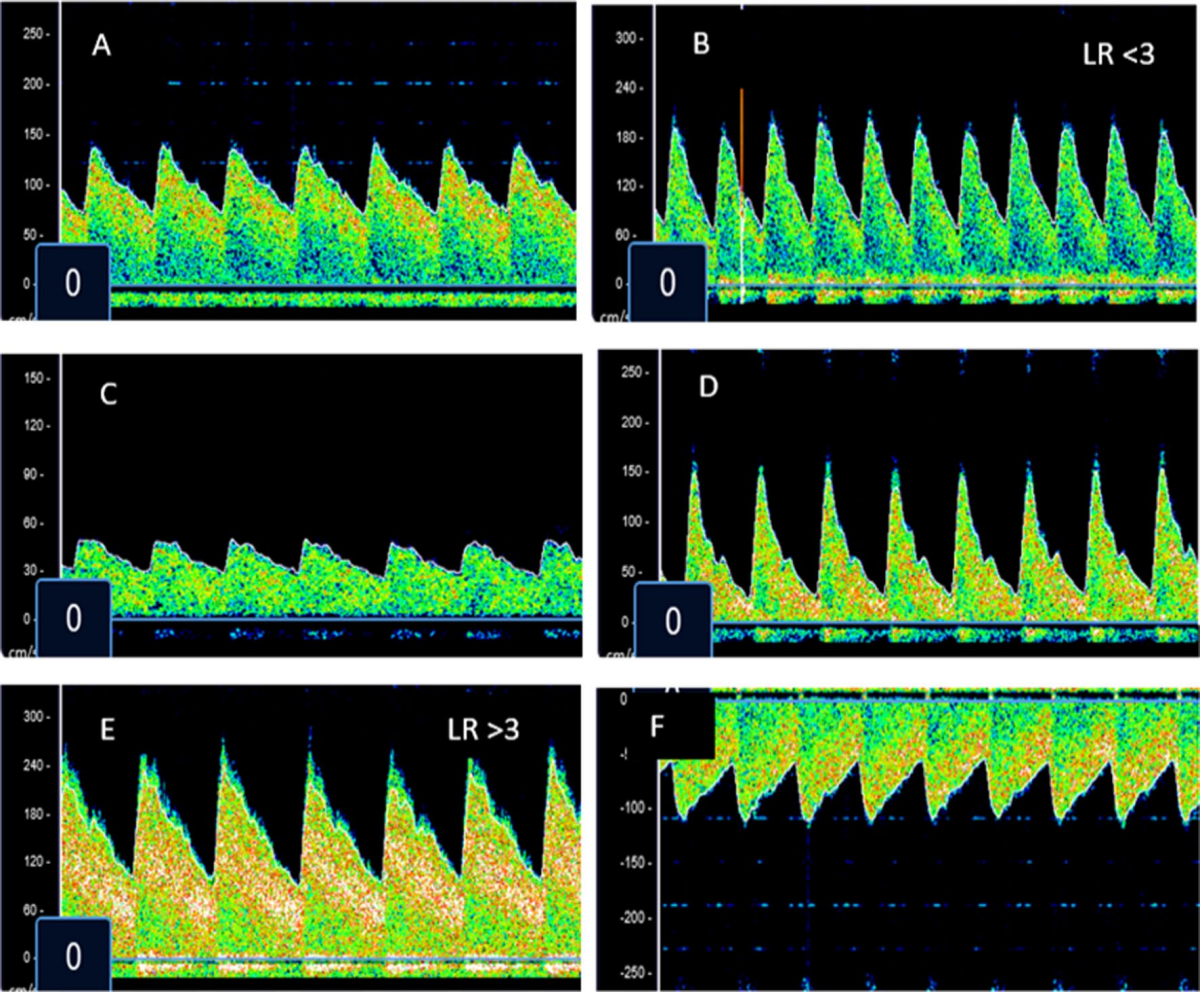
Representative images of Transcranial Doppler Ultrasound phenotypes in children with cerebral malaria. A Normal middle cerebral artery (MCA) TCD flow velocities and waveform for a 3-year-old child. B TCD with increased systolic flow velocity, increased diastolic flow velocity, Lindegaard ratio (LR) < 3. These findings represent a child categorized as having hyperaemia. C TCD with decreased systolic flow velocity, decreased diastolic flow velocity, decreased mean flow velocity. These findings represent a child categorized as having low flow. D TCD with normal systolic flow velocity, reduced diastolic flow velocity, increased pulsatility index. These findings represent a child categorized as having microvascular obstruction. E TCD with increased systolic flow velocity, increased diastolic flow velocity, LR > 3. These findings represent a child categorized as having cerebral vasospasm. F TCD with increased systolic flow velocity, increased diastolic flow velocity, increased mean flow velocity in the basilar artery. At the same time, all measurements in the MCAs were normal. These findings represent a child categorized as having isolated posterior circulation high flow
